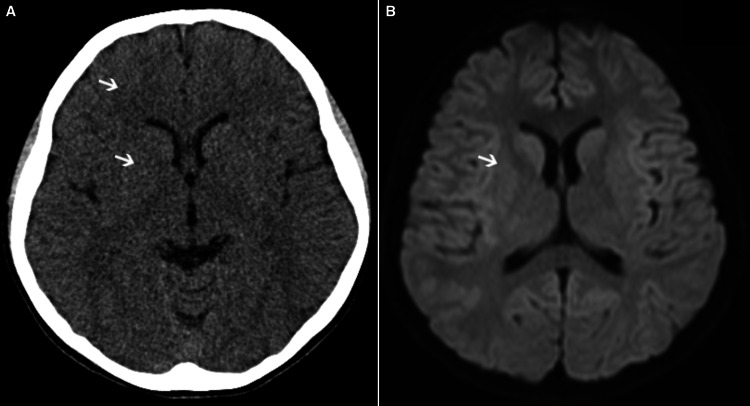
Representative image of the patient's brain (A) An initial plain CT scan. (B) Diffusion-weighted magnetic resonance imaging performed on Day 3. Both imaging showed no abnormalities. The arrows indicate that the normal structure of the brain is maintained.
